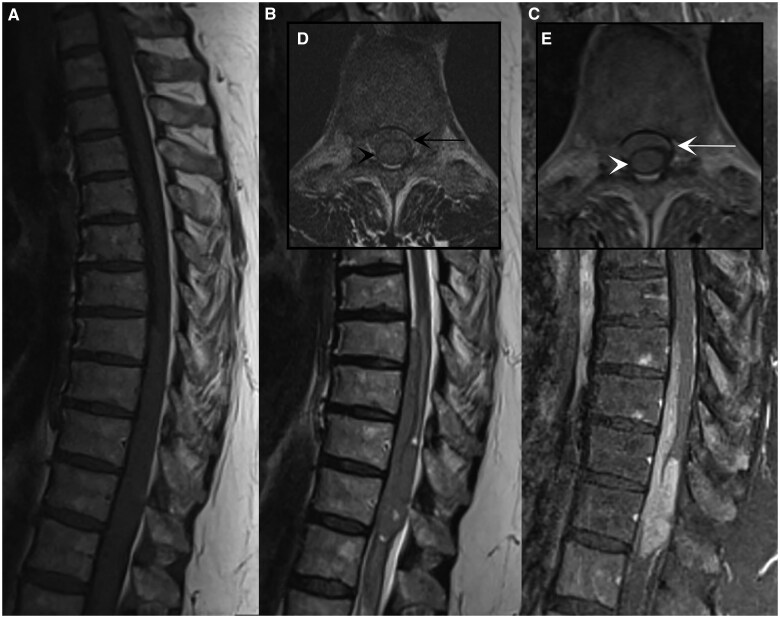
MRI of the thoracic spinal mass. (A) Sagittal T1-weighted: intramedullary and extramedullary mass is isointense to the spinal cord. (B and D) Sagittal and axial T2-weighted: mass appears mildly hyperintense to the cord with small cystic foci at the superior and inferior margins of the intramedullary component and associated cord oedema. On axial (D), there is cord expansion (black arrowhead) and a ventral extramedullary component (black arrow) which fills the thecal sac with no visible surrounding cerebral spinal fluid (CSF). (C and E) Sagittal T1-weighted fat saturation post-contrast and axial T1-weighted post-contrast: avid enhancement of both the intramedullary component (white arrowhead) and ventral extramedullary component (white arrow).
